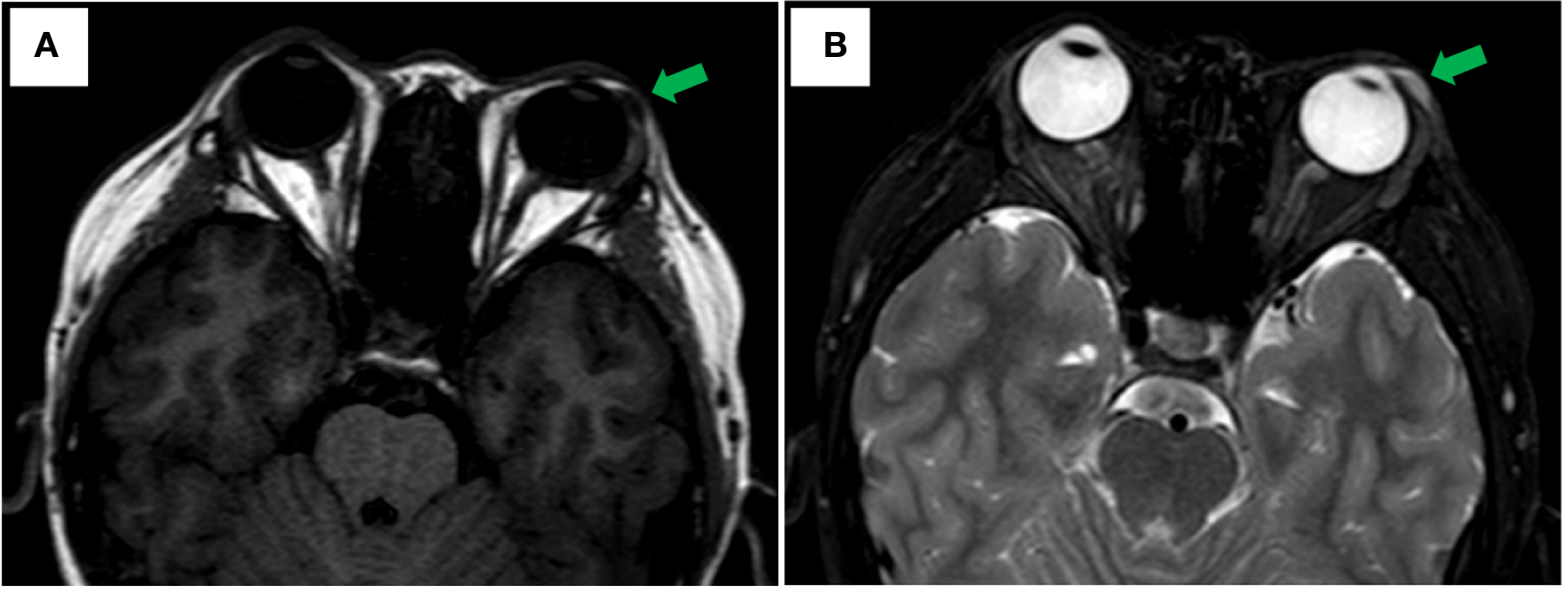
Axial MRI Imaging. (A) Well-circumscribed fusiformmass is noted in the left lower eyelid appearing hypointense on T1 weighted image with green arrowhead. (B) T2-weighted showing hyperintense with green arrowhead.
